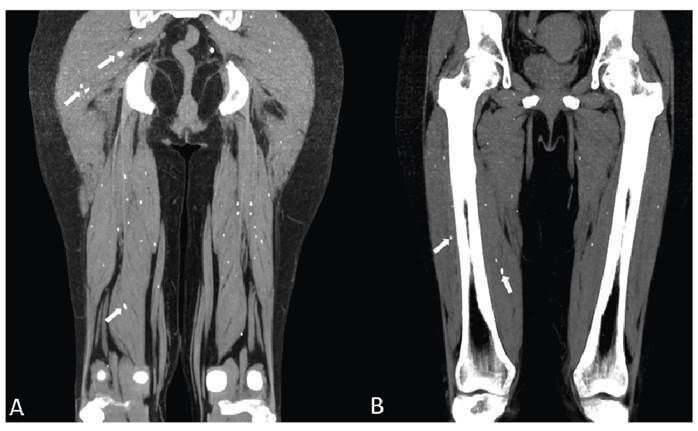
Coronal (A and B) non-contrast-enhanced computed tomography with maximum intensity projection reveals multiple elongated and oval-shaped calcified lesions within the hip and thigh muscles.
